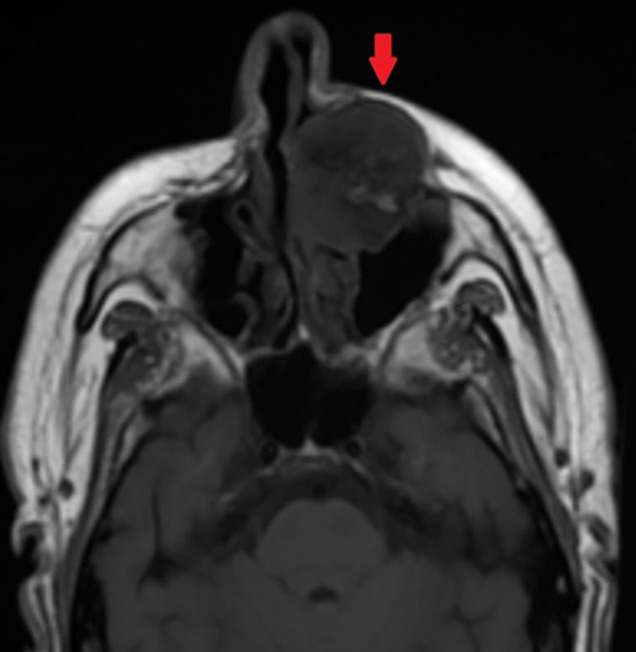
axial T1-weighted image showing a hyporintense lesion of the left maxilla (red arrow)
